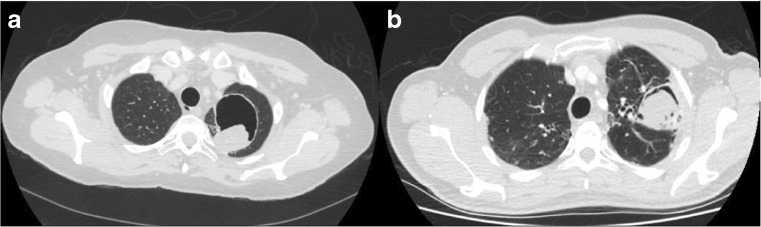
Computed tomography (CT) scan of the thorax shows a simple aspergilloma in an asymptomatic patient with a thin-walled cavity and a fungal ball in the left lung. b CCPA in a symptomatic patient with cough, shortness of breath and recurrent haemoptysis, and the image shows a left sided thick-walled cavity with pleural thickening and fungal ball
